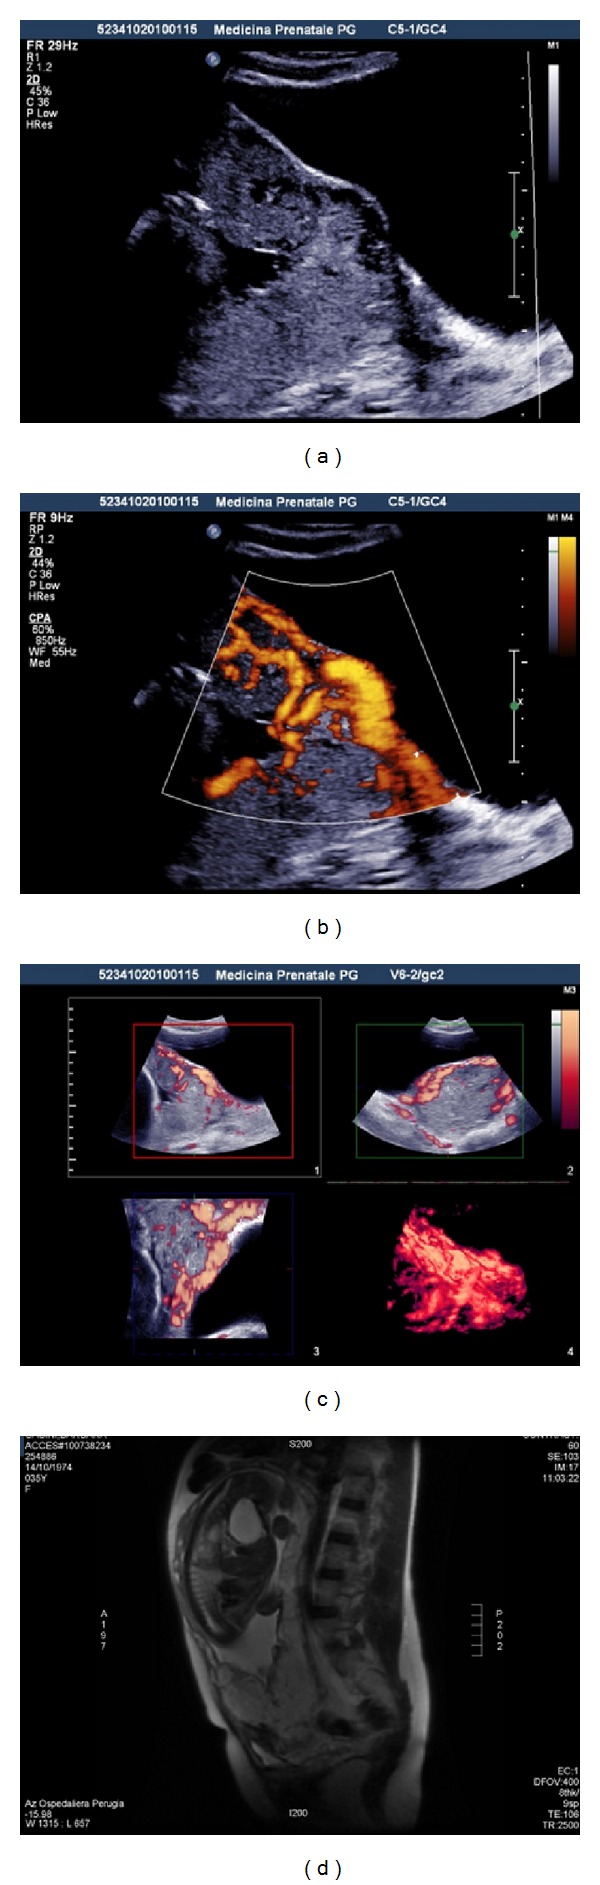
Ultrasonographic-RMN diagnosis of placenta previa accrete. (a) 2D transabdominal ultrasound showed the absence of placental- miometral interface, the uterine wall being undistinguishable from the placenta, and the presence of multiple vascular intraplacental lacunae “Swiss cheese placental appearance,” (b) 2D color Doppler ultrasound revealed an extensive vascularity along the anterior portion of the lower uterine segment and appears to extend up to and around the bladder, (c) 3-dimensional power Doppler showed the presence of numerous retroplacental vessel, and (d) pelvic magnetic resonance (MR) confirmed the ultrasound diagnosis of placenta previa accreta with partial wall bladder invasion.
